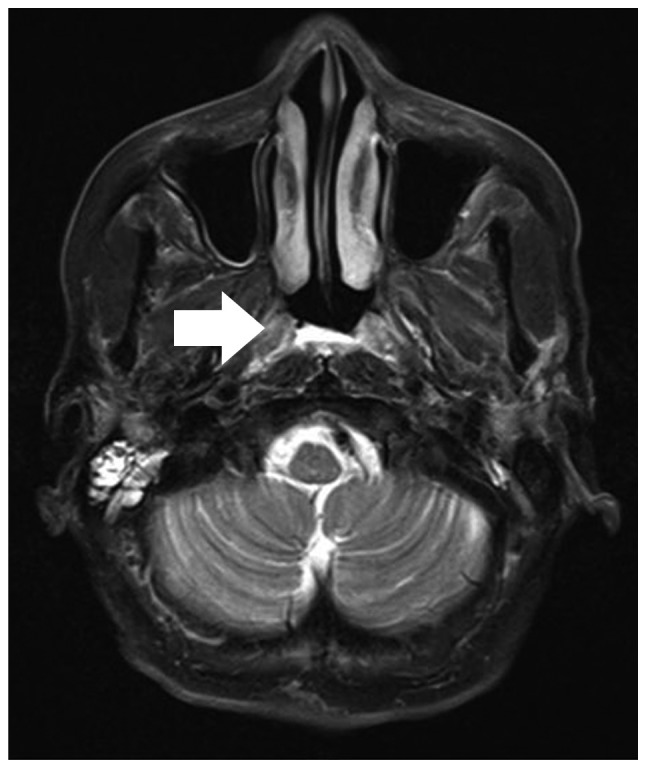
Magentic resonance imaging (MRI) at one month post-anti-tumor therapy (September, 2011) showing a decrease in the soft-tissue thickening at the roof of the nasopharynx (arrow) (compared with results from the MRI performed in February 2011).
